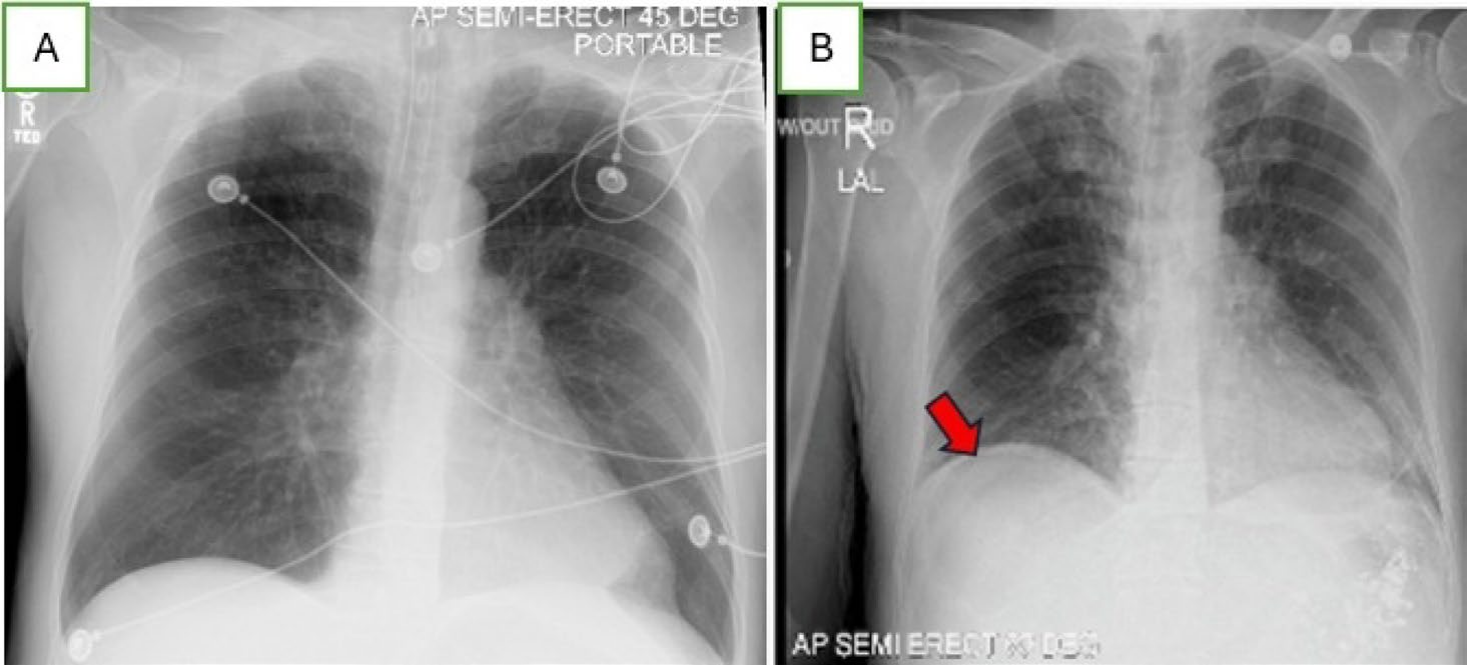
X‐ray of the chest and abdomen. (A) Anteroposterior chest radiograph obtained prior to extubation and before barium swallow evaluation. (B) Semi‐erect chest film showing free air under the right hemidiaphragm (red arrow).
